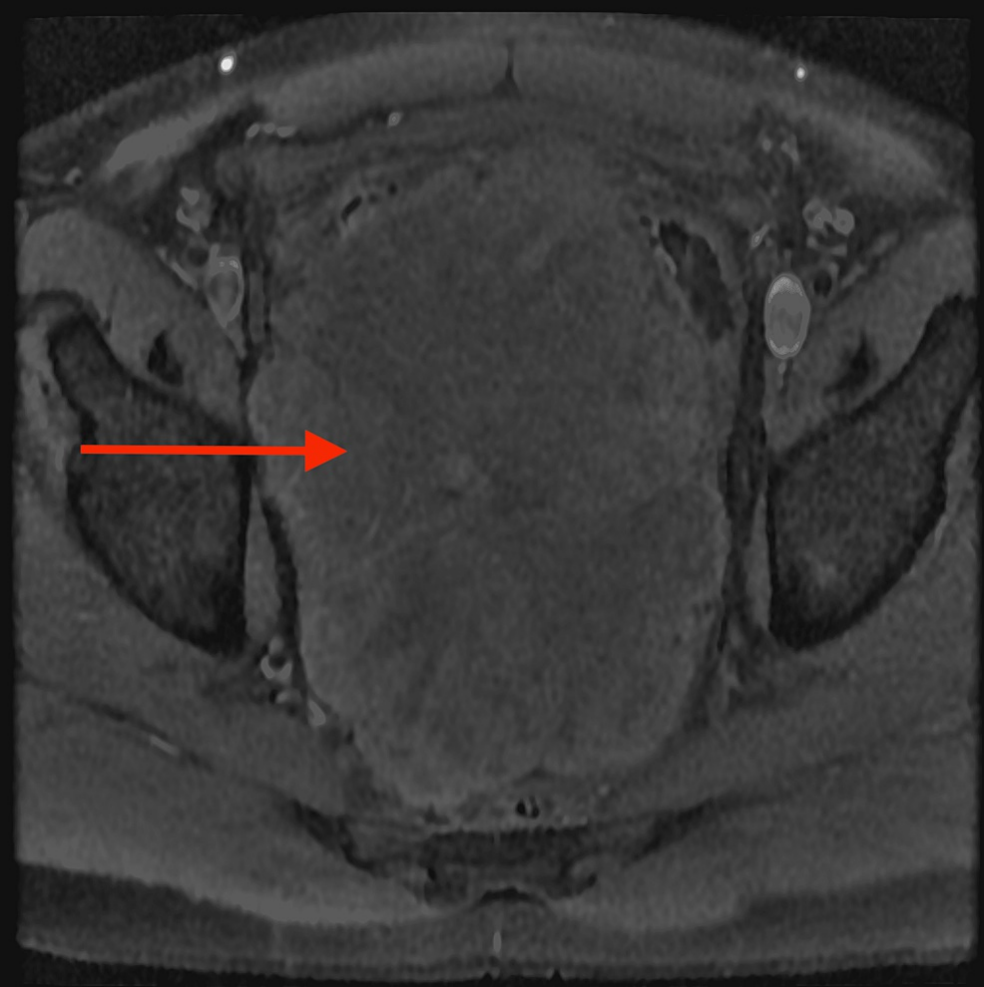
Axial MRI T1 pre-contrast image demonstrates a large lobulated mass (red arrow)
